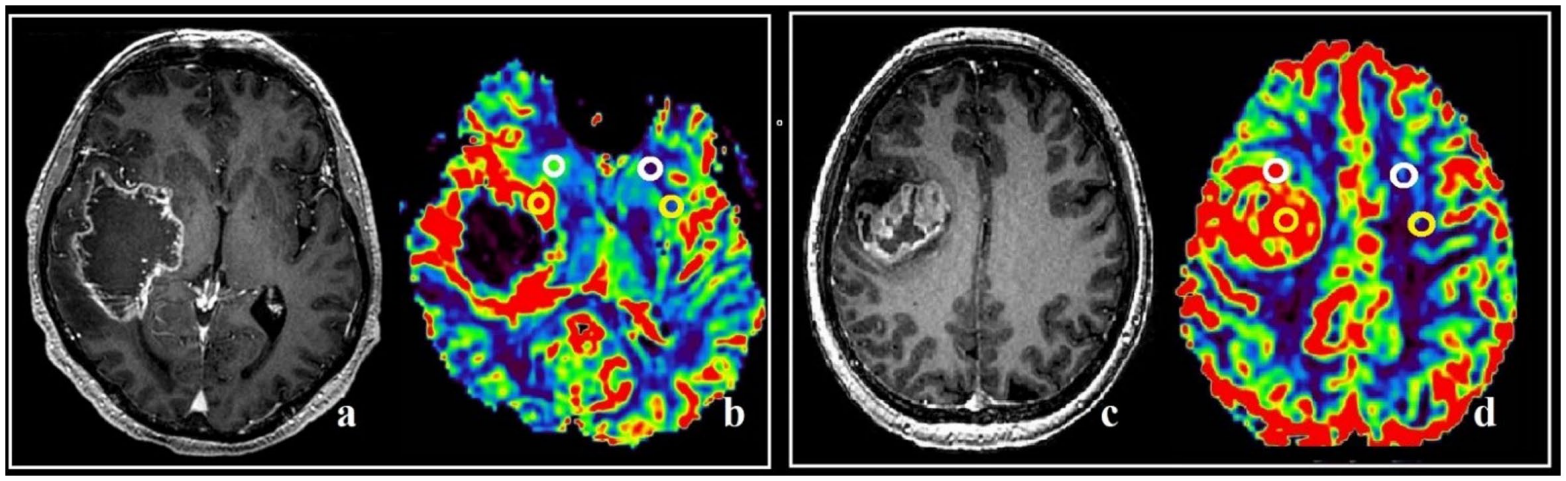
Post-contrast T1 WI and MRI, and CT-perfusion of different patients (a–d). ROI segmentation with altered (yellow circles) and intact (white circles) BBB during comparison with post-contrast T1 series and DSC-T2* perfusion CBV map (a,b), and post-contrast T1 series with CT-perfusion CBV map (c,d). The parameters analysis was conducted both in affected and in specular contralateral hemisphere white matter.
